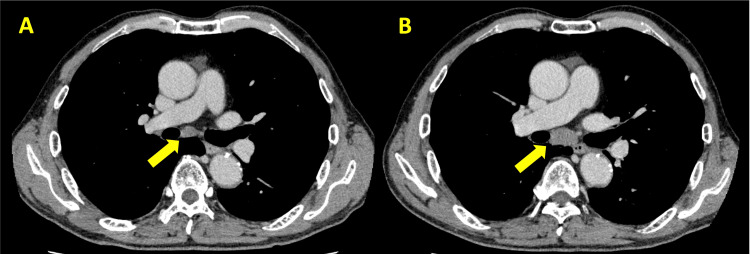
Progressive enlargement of mediastinal lymph nodes on contrast-enhanced CT A: initial axial contrast-enhanced computed tomography (CT) image of the chest showing mild mediastinal lymphadenopathy (yellow arrow) without significant compression of adjacent structures; B: follow-up axial contrast-enhanced CT image of the chest demonstrating progressive enlargement of the mediastinal lymph node (yellow arrow) compared to image (A). This increase in size raised suspicion of malignancy, leading to further evaluation via EBUS-TBNA. EBUS-TBNA: endobronchial ultrasound-guided transbronchial needle aspiration
